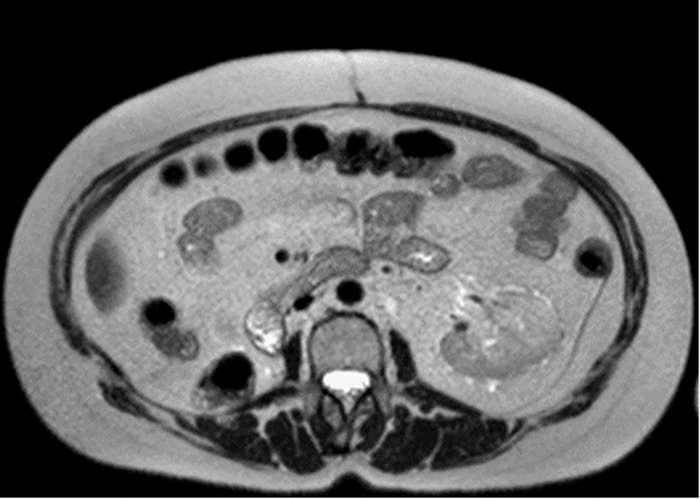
T2-weighted axial magnetic resonance image shows complete response to stereotactic body radiotherapy.
